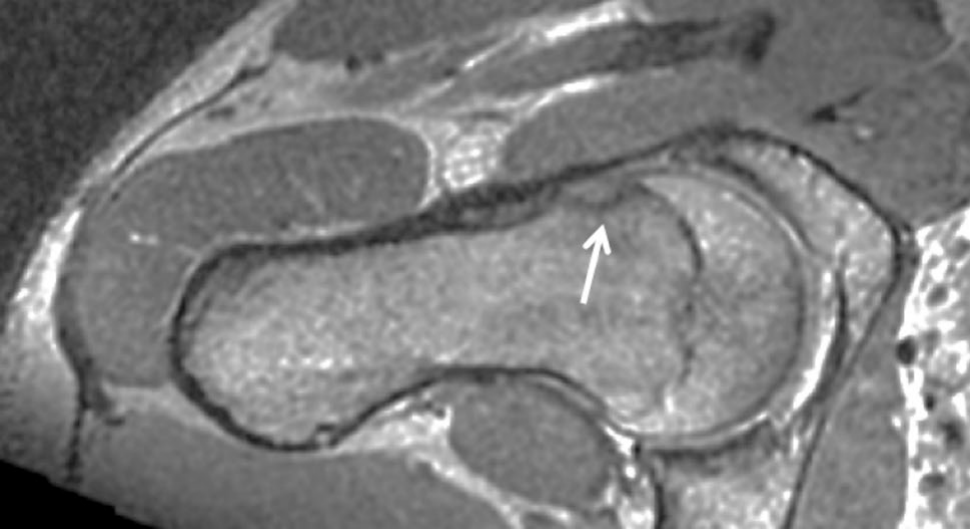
MRI examination of a male skier showing cam morphology with an *α*-angle above > 55° (arrow)
